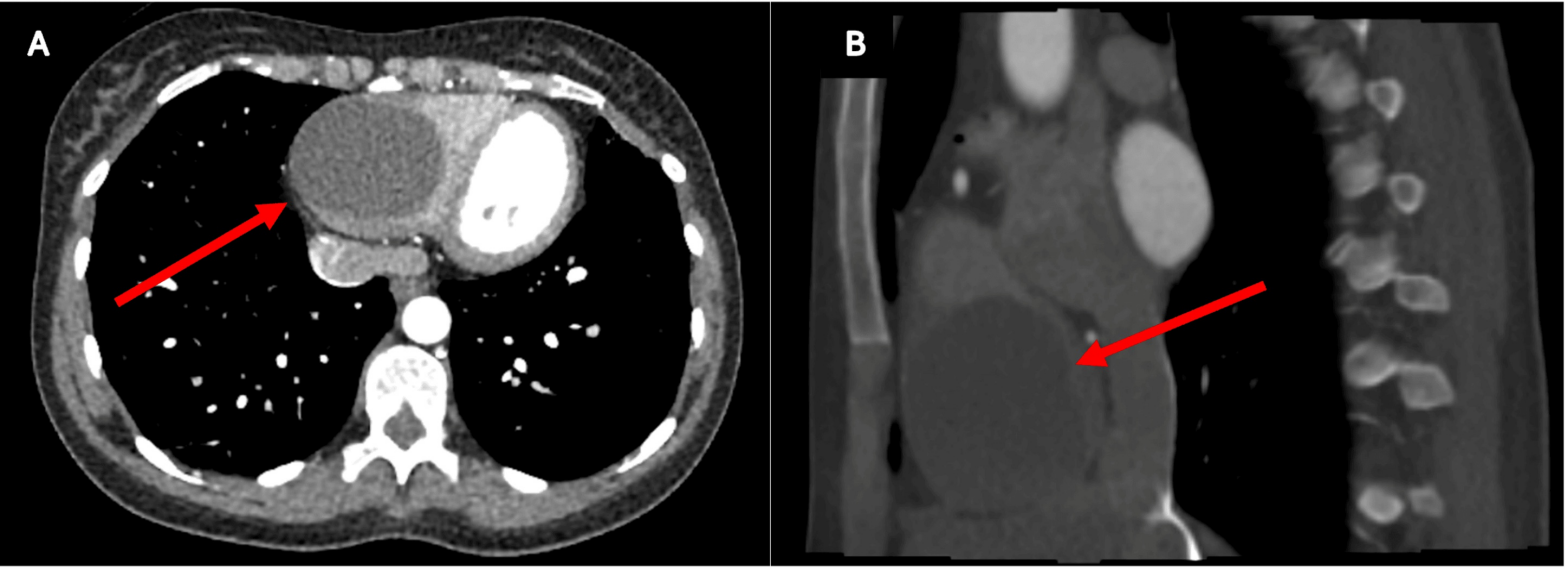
Preoperative CT scan showing a right ventricular mass. Images (A) and (B) show the transverse and sagittal views of the mass, respectively. CT, Computed tomography
